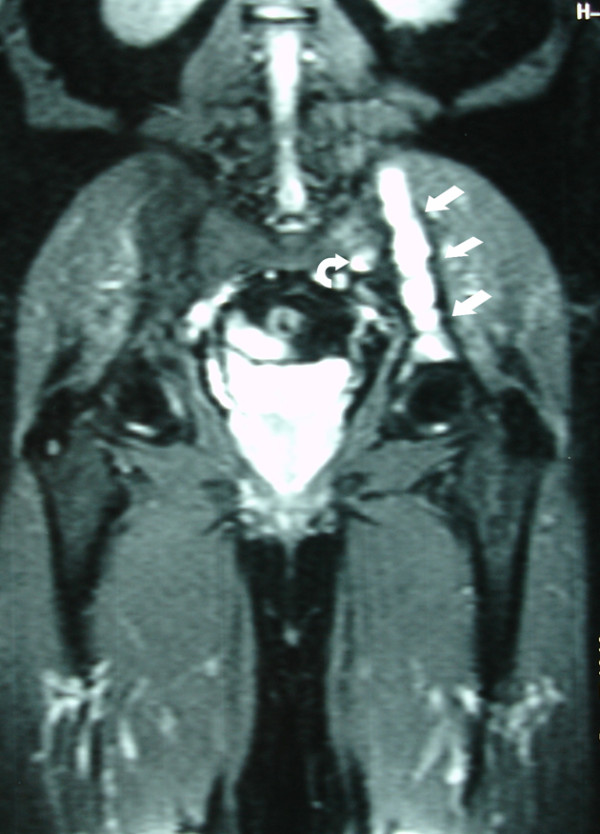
Coronal STIR.MRI image shows an increased signal at the left iliac bone (arrows) and at the left sacral wing (curved arrow).
